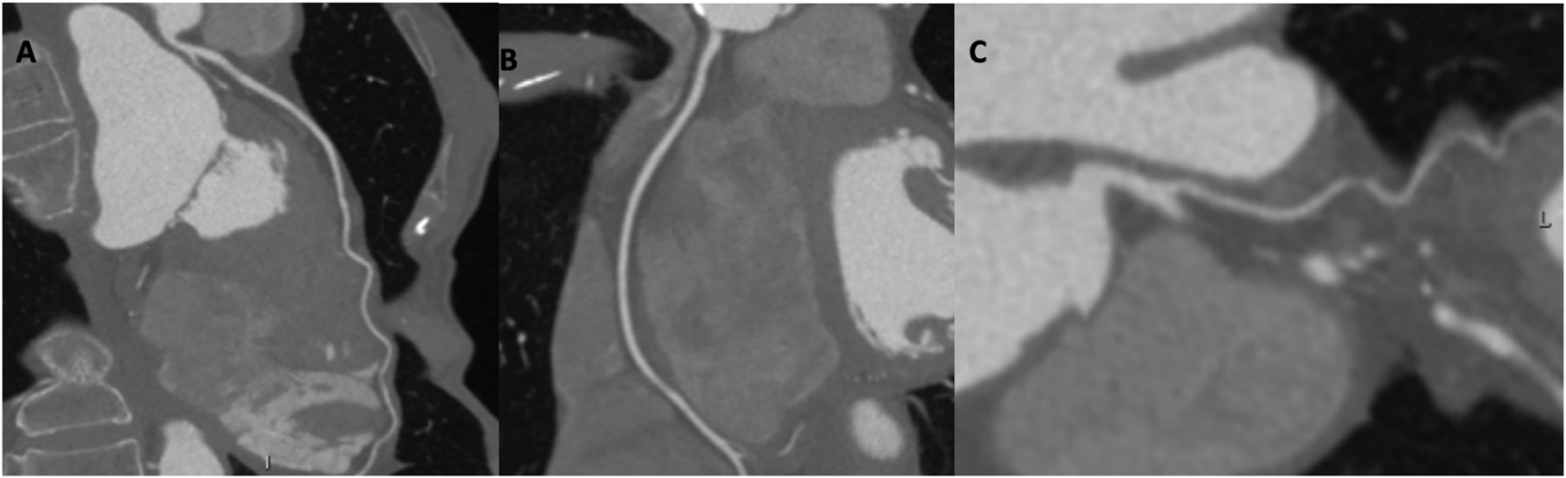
64-year-old female patient underwent to coronary computed tomography angiography for dispnea. The left main and left anterior descending artery showed no atherosclerosis (A) as well as right coronary artery (B) and circumflex (C).
